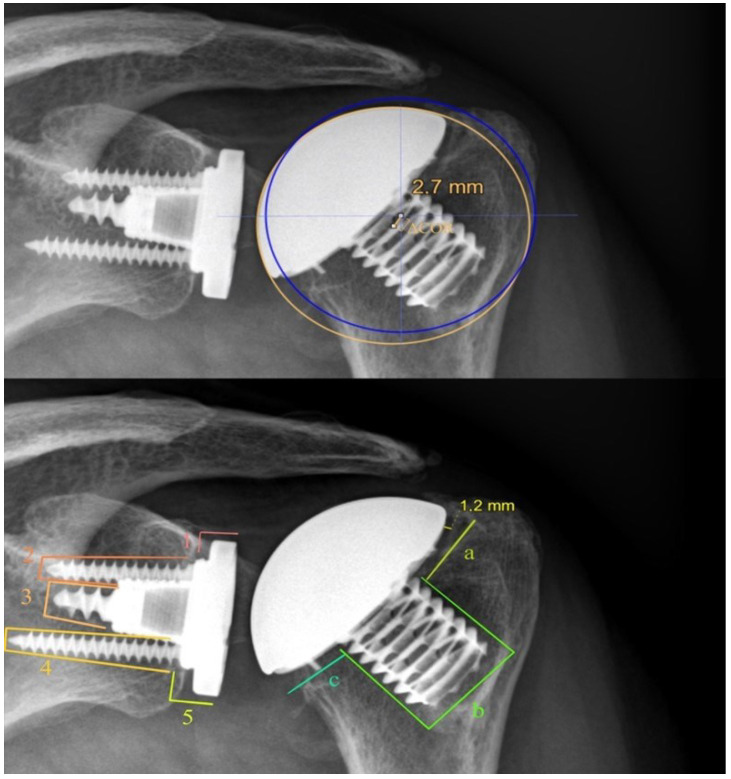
Postoperative radiographs of the left shoulder. The above X-ray image shows the anatomical circle (blue) and the implant-matched circle (orange), according to Alolabi et al. [11]. The distance between the two centers was measured (ΔCOR). In the X-ray image below, an assessment of radiolucent lines (RLs) for the glenoid and humeral components is shown. Glenoid RLs were quantified in 5 zones (1–5) considering their thickness, while the humeral RLs were quantified in 3 different zones (a, b, and c). The Radiograph shows radiolucency in zone a.
